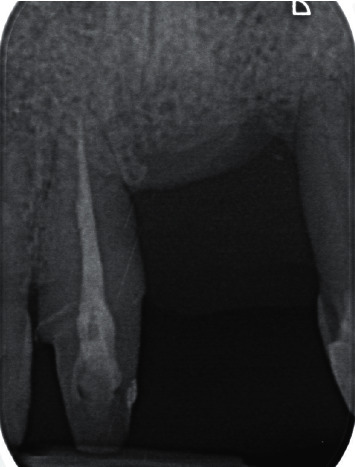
Final intraoral periapical radiograph.
